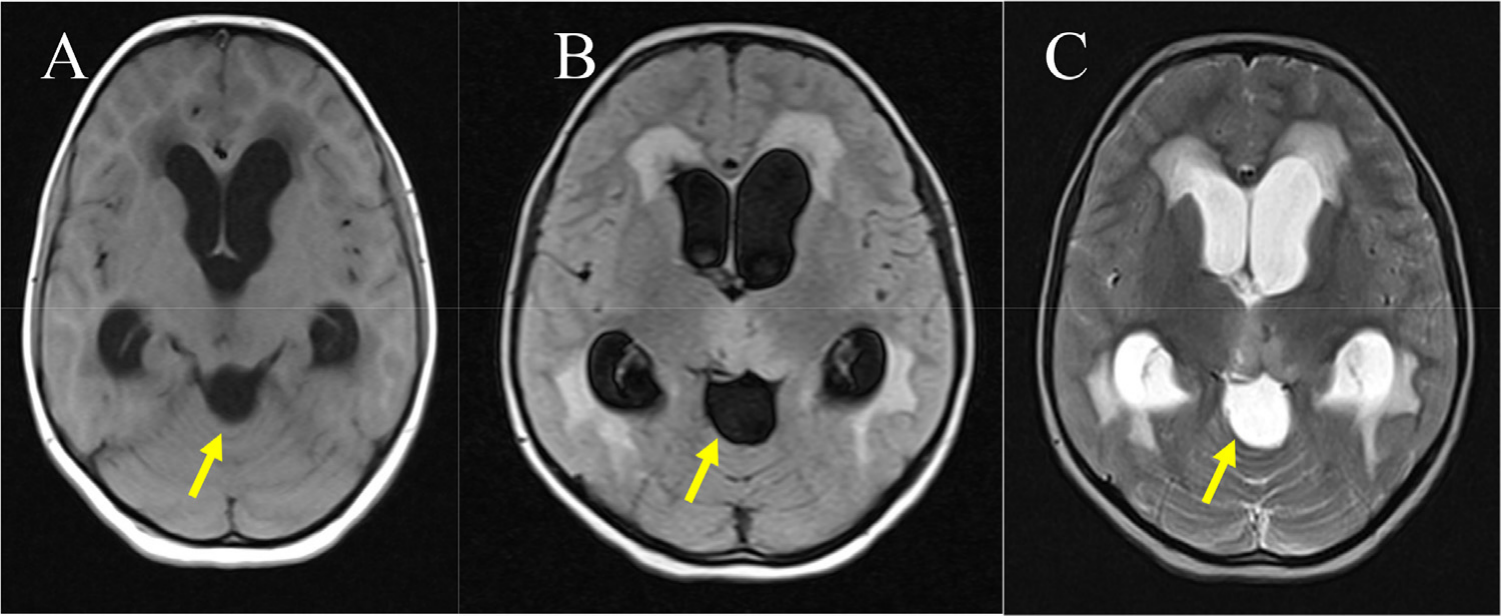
Noncontrast Axial MRI shows a well-demarcated, homogenously cystic mass (arrows) occupying the quadrigeminal cistern and following cerebrospinal fluid (CSF) signal intensity on T1-weighted (A), FLAIR (B), and T2-weighted images (C).
